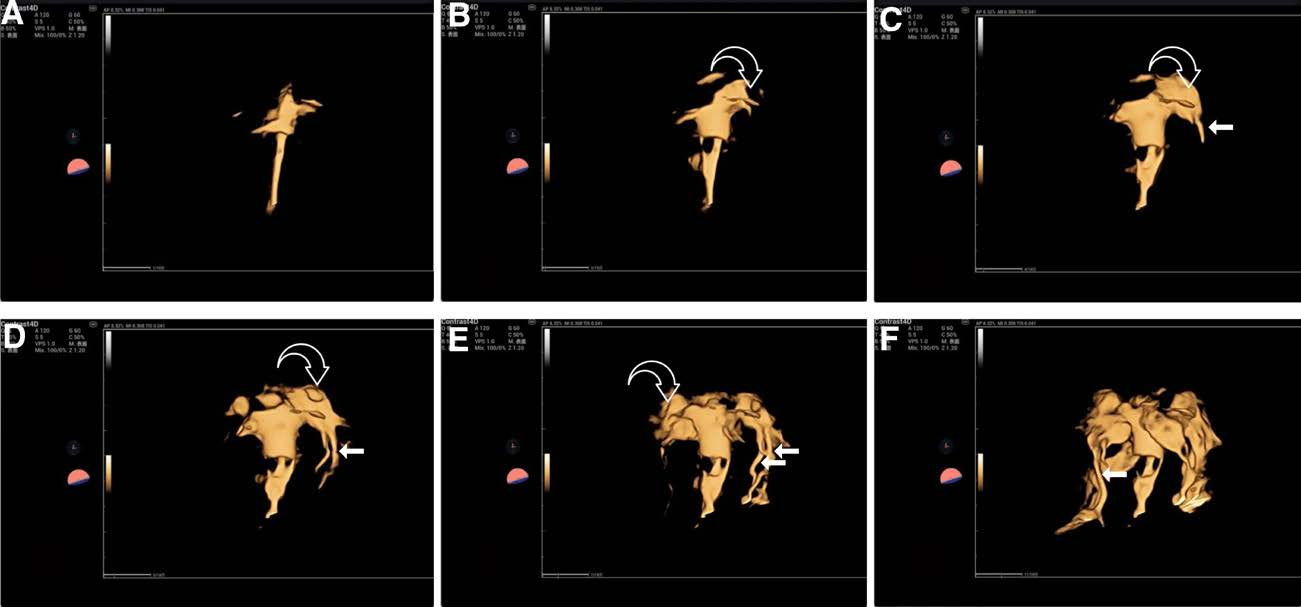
4D HyCoSy mode: (A) the contrast agent enters the uterine cavity along the contrast catheter. (B) The left uterine horn begins to visualize, but its morphology is abnormal (the white hollow arrows). (C) The contrast agent further fills and distends the left uterine horn (the white hollow arrows), with a tubular structure visible at its distal end (the white solid arrows). (D) Two tubular structures are visible at the margin of the distended left uterine horn (the white solid arrows). (E) The left tubular structure continues to extend, showing an appearance highly like that of a fallopian tube-like structure (the white solid arrows); meanwhile, the myometrium of the right uterine horn exhibits abnormal visualization and distension (the white hollow arrows). (F) A fallopian tube-like structure is visible at the margin of the distended right uterine horn, which is like the left tubular structure (the white solid arrows). 4D = 4-dimensional, HyCoSy = hysterosalpingo-contrast sonography.
